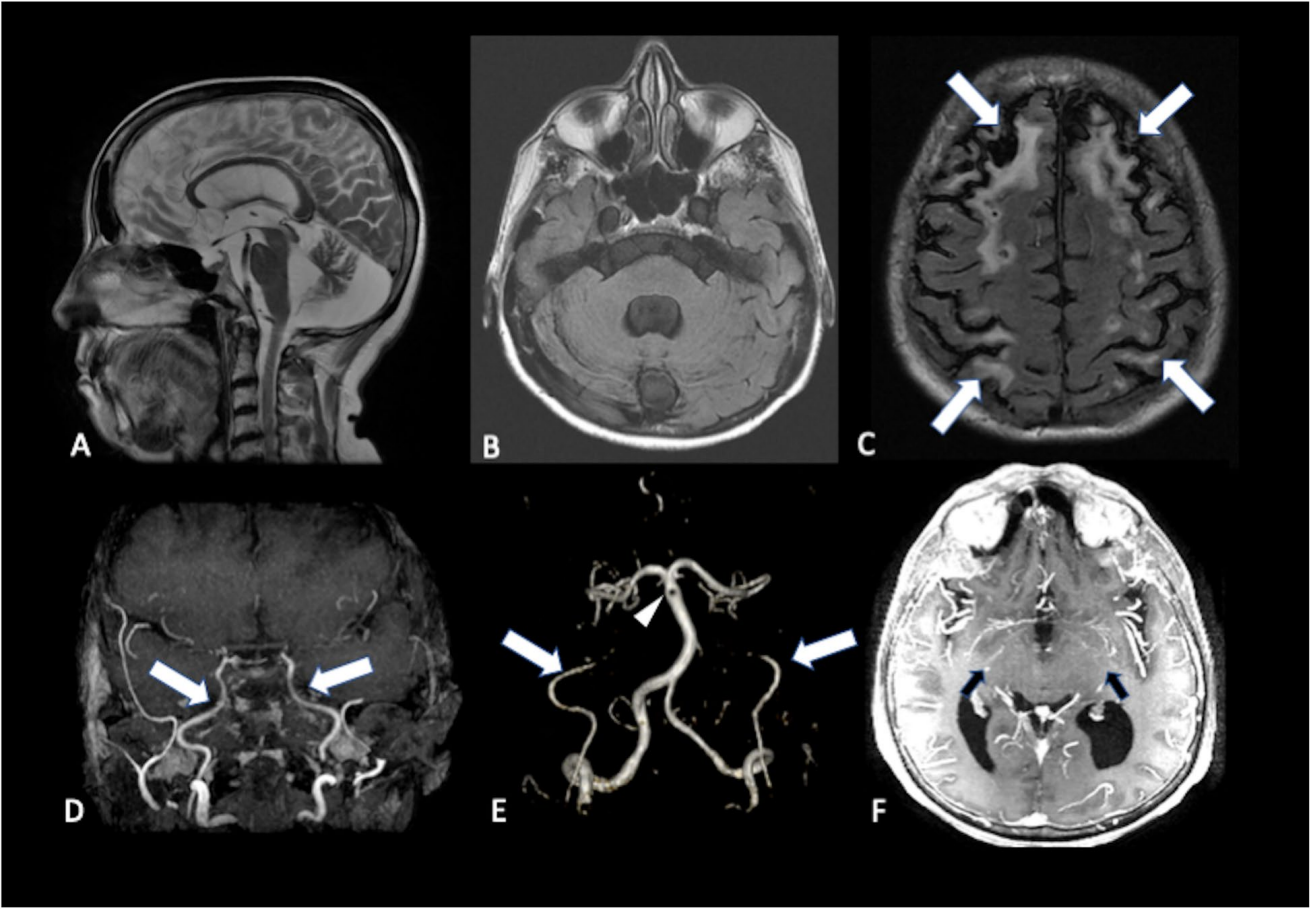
Sagittal T2 (A) and axial FLAIR (B) images showing cerebellar atrophy and (C) chronic watershed zone infarcts (arrows). MR angiography reveals progressive bilateral intracranial carotid narrowing, followed by occlusion in supraclinoid segments (D), absence of flow signal in both supraclinoid carotid segments (E) and abnormal net-like vessels resembling a "puff of smoke” (F)
